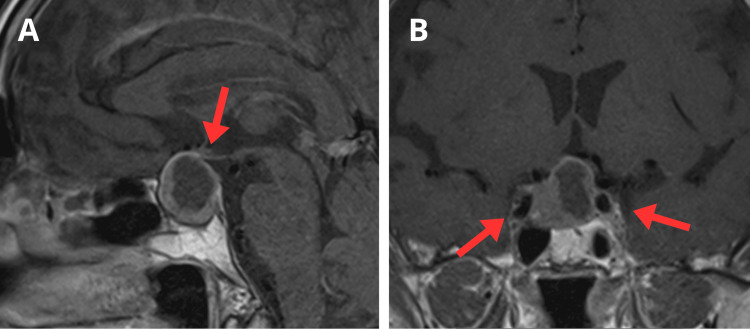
Preoperative magnetic resonance imaging of the pituitary gland Sagittal T1-weighted gadolinium-enhanced MRI (A) and coronal MRI (B) demonstrating a cystic pituitary macroadenoma, suggestive of apoplexy. The tumor exhibits suprasellar extension, compressing the optic chiasm (A), and invasion of the right cavernous sinus (Knosp grade 2) (B).
